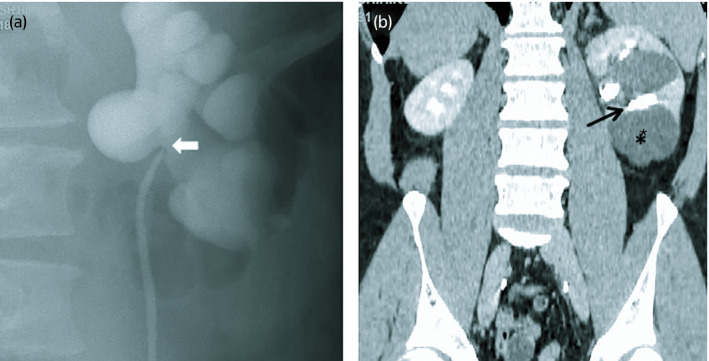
(a) Retrograde pyelogram shows left hydronephrosis with high ureteral insertion and intrarenal UPJO area (arrow). (b) Contrast‐enhanced CT‐KUB in the delayed phase. The left lower calyx (arrow) is adjacent to a renal cyst (*).
